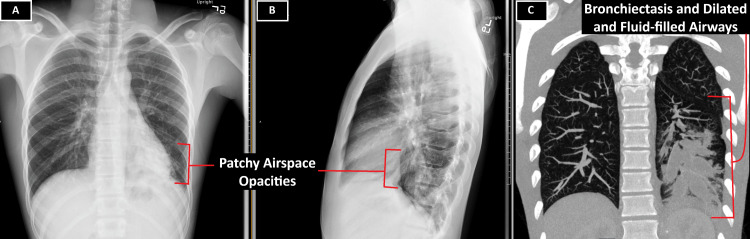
Chest X-ray and CT chest with contrast A and B: The patient's AP and lateral chest X-rays revealed patchy airspace opacities within the left lower lobe, thought to represent pneumonia. C: The patient's CT chest with contrast showed bronchiectasis and dilated and fluid-filled airways can be visualized throughout the left lower lobe.
